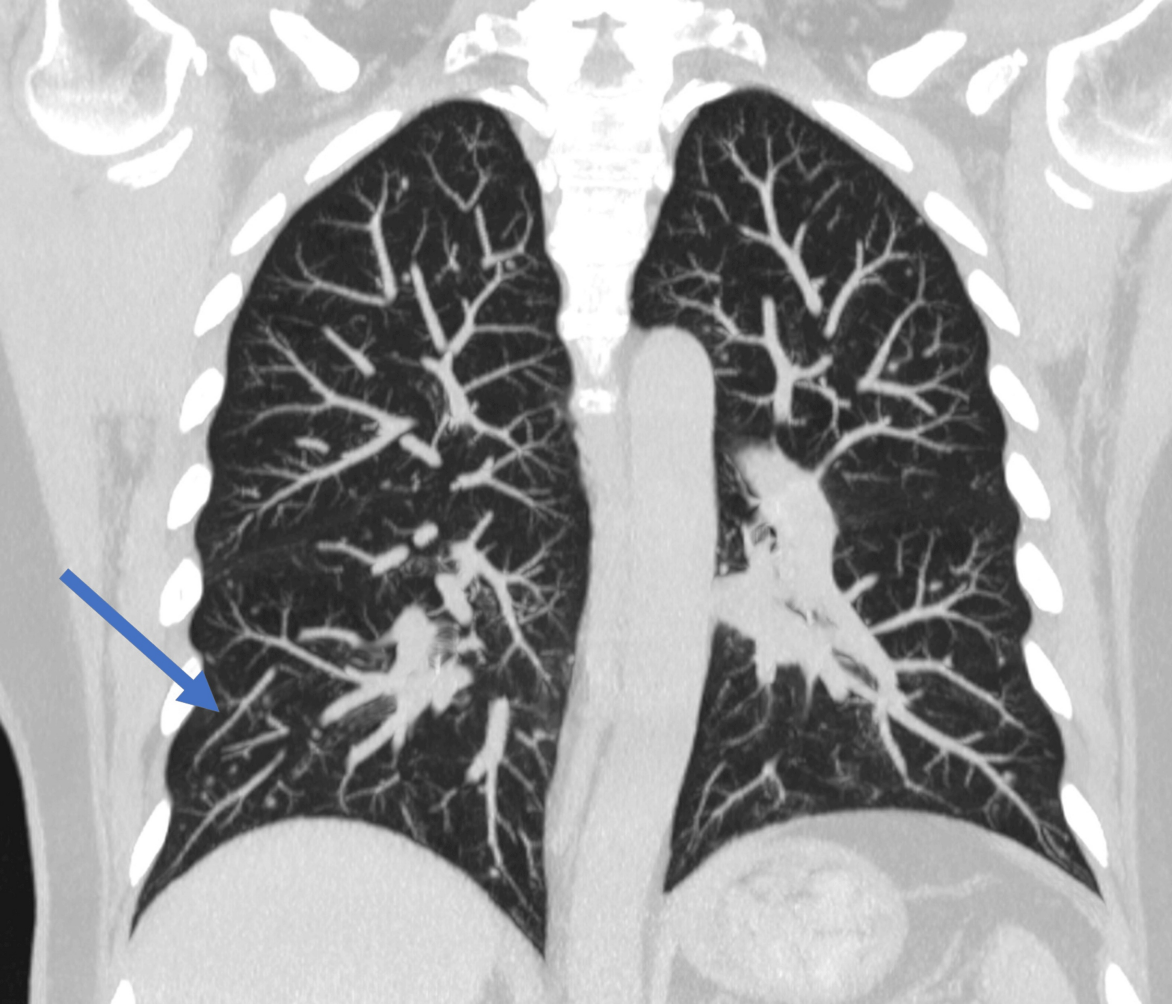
Chest CT coronal plane MIP (maximum intensity projection) performed eight weeks after symptoms shows a significant reduction in bilateral solid nodules (blue arrow).
